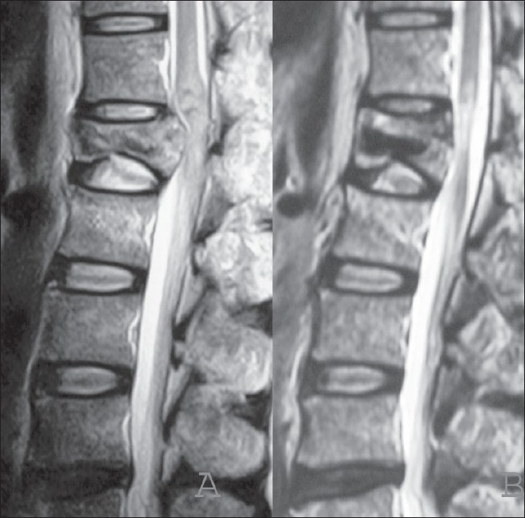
(A) Preoperative T2WI mid sagittal MR imaging showing severe compression of the dural sac by bone retropulsion. Three-year postoperative sagittal MR imaging (B) demonstrating decompression of the spinal canal and signal intensity regression of the disc above the fractured vertebra
